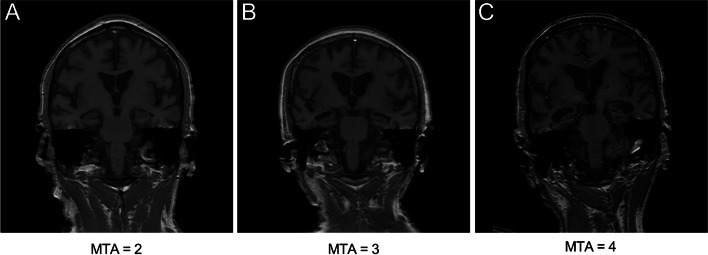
Representative MRI of MTA score rating. Representative T1-weighted images in the coronal plane depicting MTA scores of 2 (A), 3 (B), and 4 (C). MRI, magnetic resonance image; MTA, medial temporal lobe atrophy
